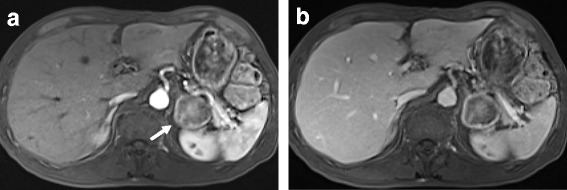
Magnetic resonance imaging (July 2009). Gadolinium-enhanced T2-weighted magnetic resonance imaging (a, early phase) indicated altered intensity in a 4-cm left adrenal tumor (*arrow*), and an area of high intensity was found along the periphery of the tumor, which persisted until the delayed phase (b)
